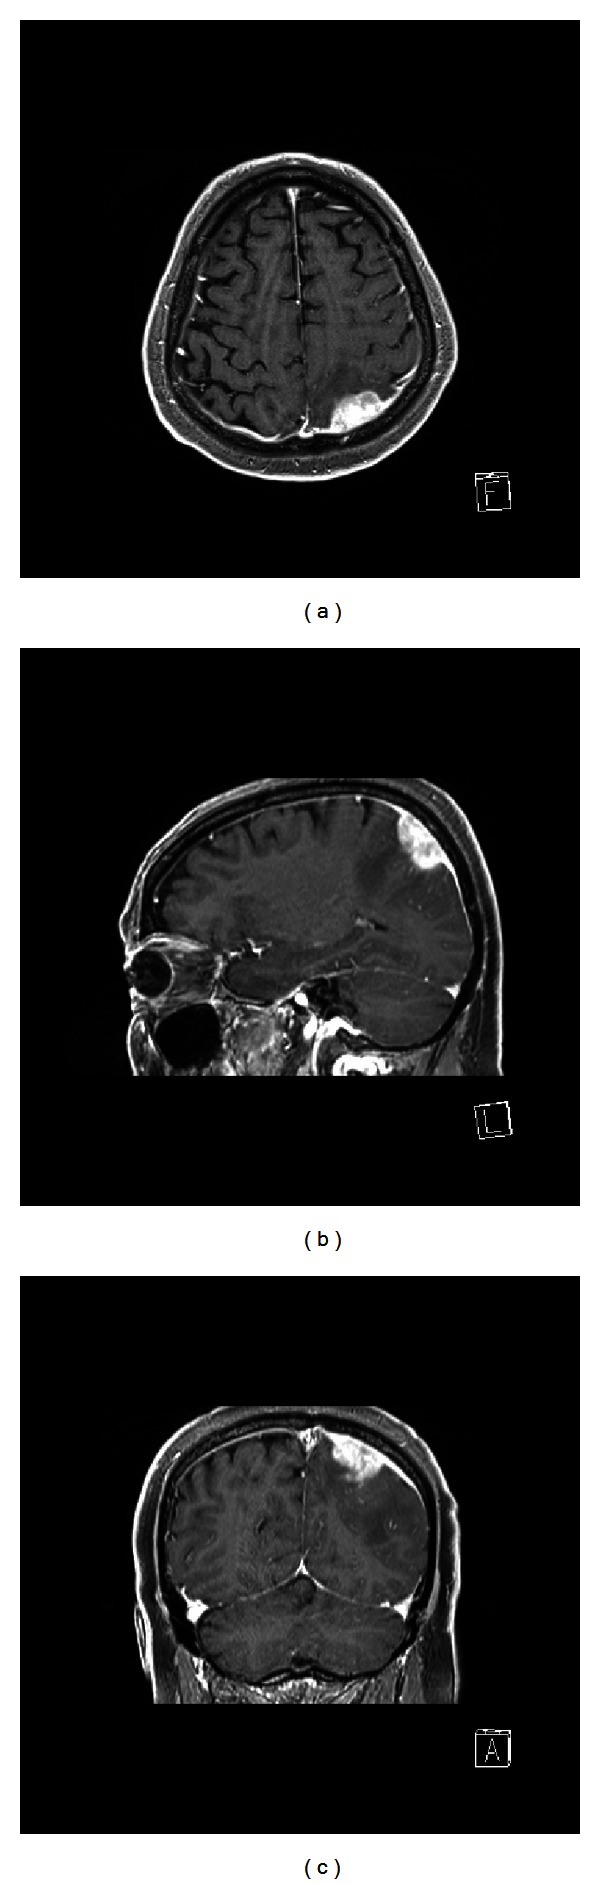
Gd-enhanced, T1-weighted MRI: axial (a), sagittal (b), and coronal (c) view of the brain granuloma; differential diagnosis with other extra-axial lesions is challenging.
